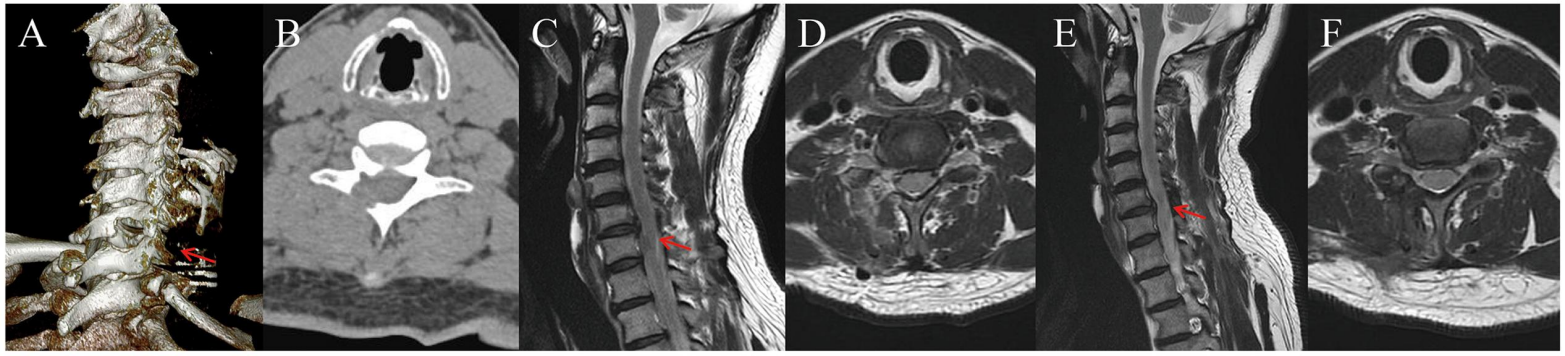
Postoperative and follow-up imaging findings. (A) Three-dimensional CT reconstruction on postoperative day 3 demonstrating adequate laminotomy and bony decompression at the C6/C7 level (arrow); (B) axial CT image confirming complete canal clearance without hematoma, bone fragment, or malalignment; (C) sagittal T2-weighted MRI on postoperative day 3 showing full decompression of the right C7 nerve root and an intact annular closure with mild localized hyperintensity at the suture site (arrow); (D) axial T2-weighted MRI depicting resolved lateral recess compression and absence of residual disc material; (E) three-month follow-up sagittal MRI demonstrating sustained neural decompression and preserved annular contour (arrow); (F) axial follow-up MRI confirming a healed annular repair site with no evidence of recurrent herniation or epidural fluid collection.
